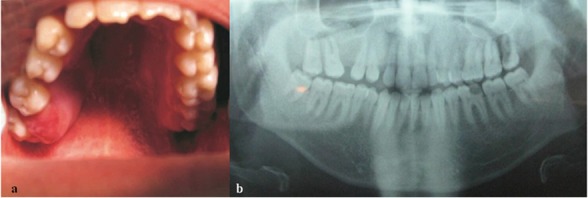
a: The photograph showing the tumor in the palatal side of left maxillary second and third molars b: Normal view in panoramic radiograph
